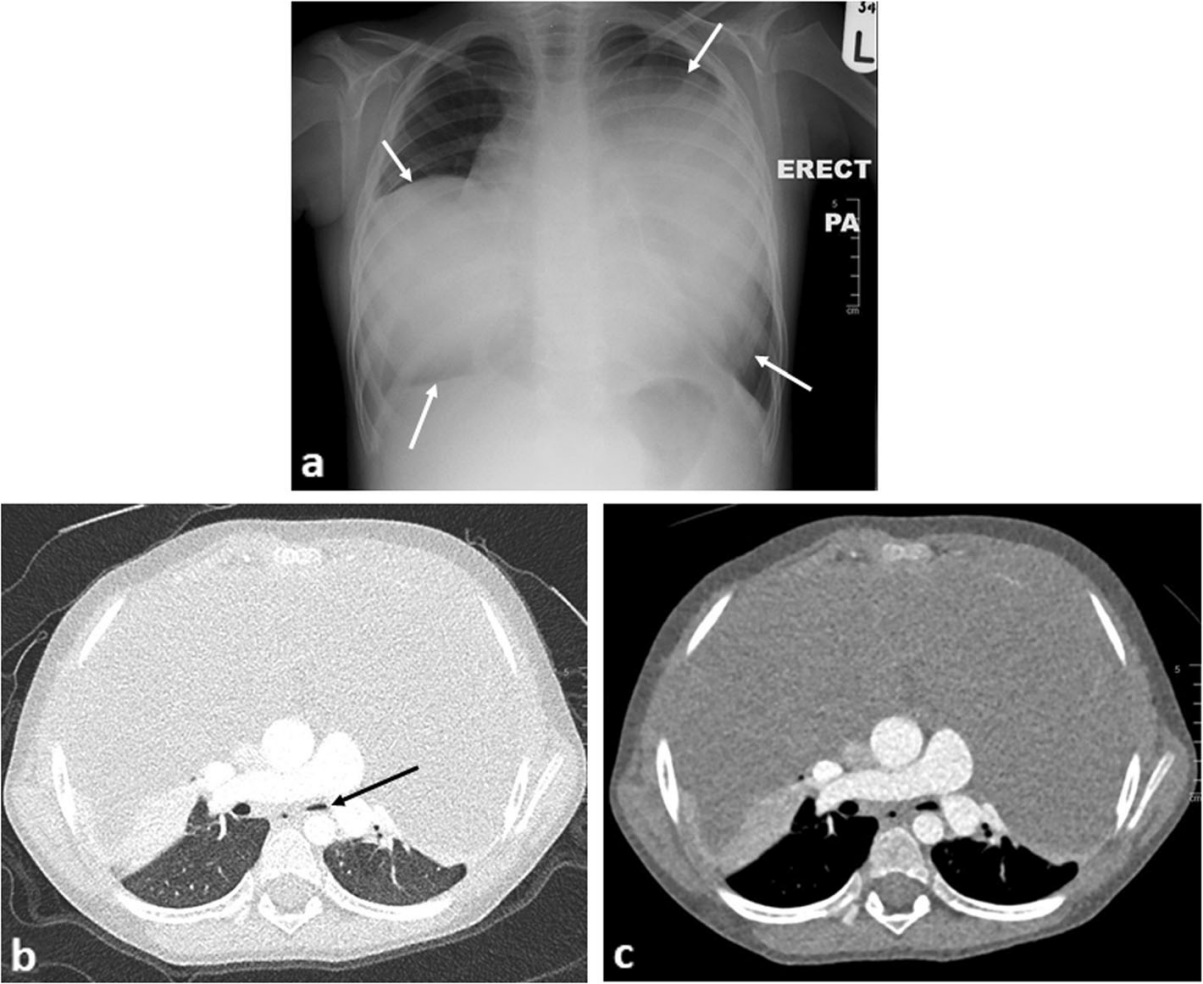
A 4-year-old boy presenting with shortness of breath secondary to desmoid fibromatosis (a benign, non-cancerous tumour). a The erect chest radiograph demonstrates an enlarged anterior mediastinum with bilateral lobular mass-like appearances (arrows). b Axial contrast-enhanced CT of the chest (lung windows) demonstrates some compression of the left lower lobe bronchus (black arrow), with patency of the right lower lobe bronchus. c Axial CT image of the chest (mediastinal windows) demonstrates the homogenous nature of the anterior mediastinal mass (which would be unusual in a suspected teratoma). In contrast to the previous figure, this patient was able to lie flat in the scanner for their imaging study despite the seemingly larger sized mass on imaging. This demonstrates how imaging findings may not necessarily relate to the patient’s symptoms and careful assessment and history remain vital
